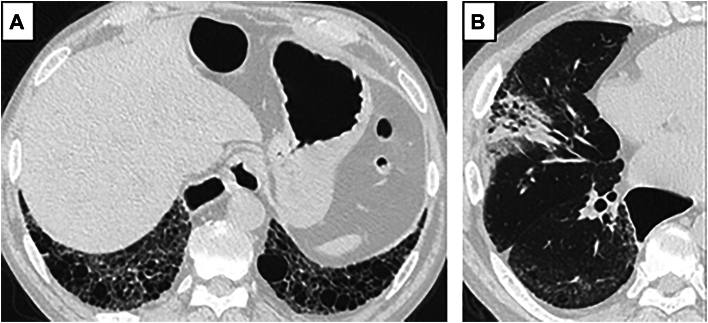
Computed tomography shows (A) a nonspecific interstitial pneumonia pattern and (B) a partially solid tumor in the right middle lobe.
